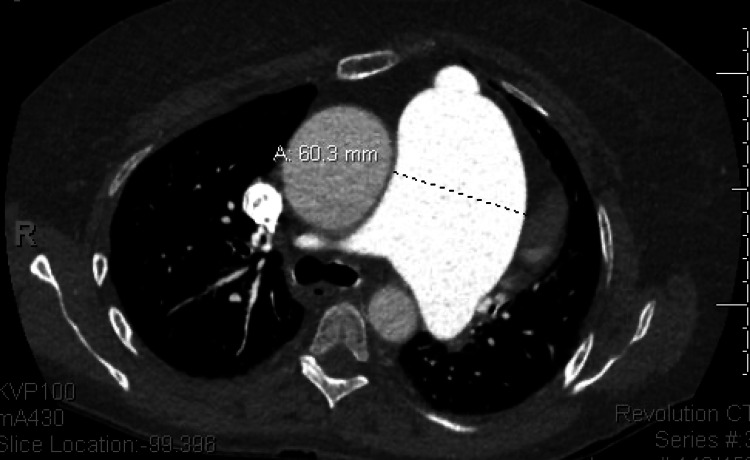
CT thorax with contrast showing a PAA measuring 60.3 mm (6.3 cm) PAA: pulmonary artery aneurysm
